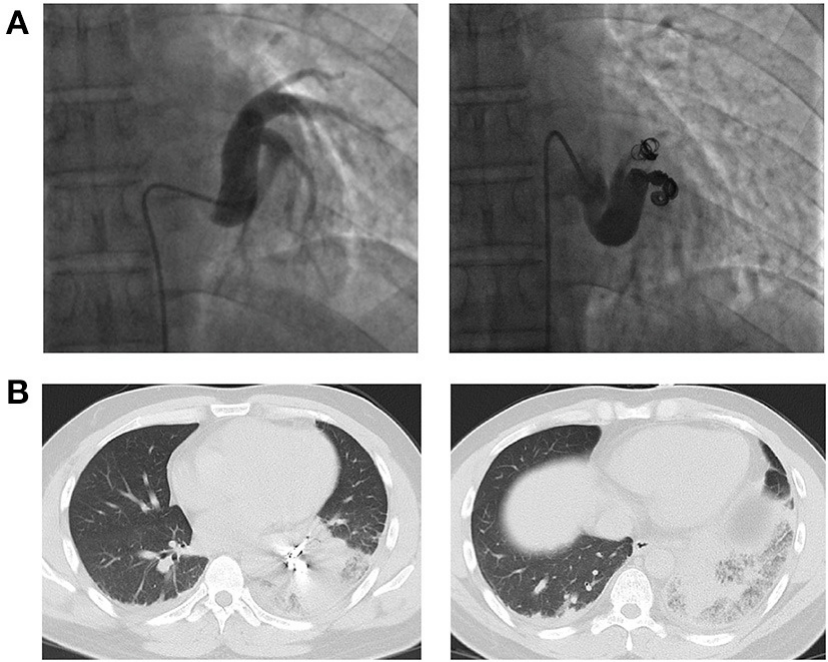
(A) Angiography and coil embolization for the anomalous systemic artery. (B) CT images showing pulmonary infarction at 48 h after the intervention.
